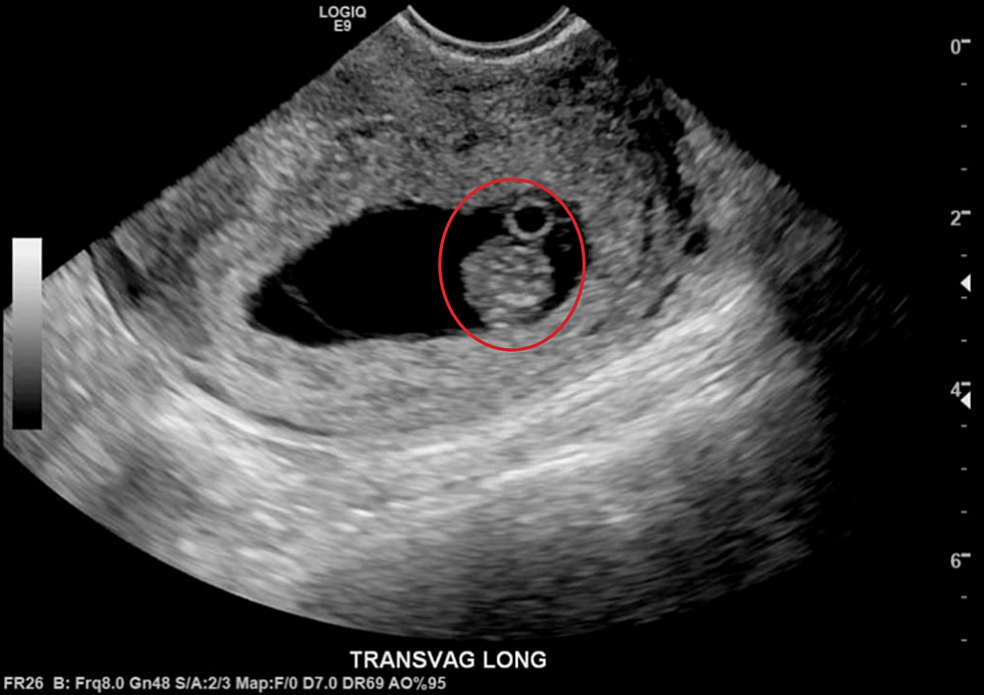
Transvaginal ultrasound showing the longitudinal view of uterus revealing intrauterine gestational sac with single fetal pole and yolk sac
